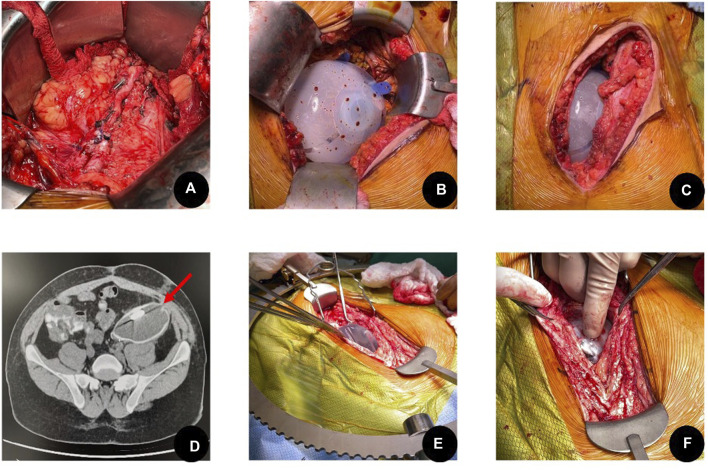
Intraoperative images of the insertion and removal of a breast tissue expander in the left iliac fossa. (A) Completed allograft nephrectomy in the left iliac fossa, highlighting the pelvic vessels ready for repeat transplant procedure, (B) Insertion of the tissue expander and appropriate sizing with instillation fluid, (C) Closure of allograft nephrectomy wound around tissue expander, (D) Pre-transplant CT scan demonstrating the implantation of the tissue expander in the left iliac fossa. (E,F) Removal of the tissue expander during the retransplantation procedure, highlighting the pseudocapsule created and ease of extraction.
